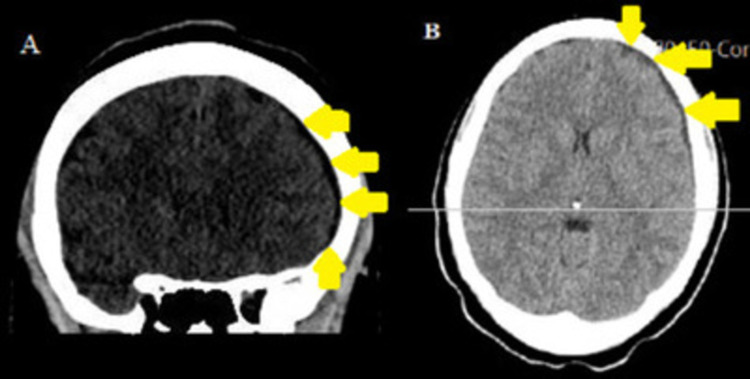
Left subdural hematoma Noncontrast coronal (A) and axial (B) CT scan of the brain demonstrating 4 mm thick subdural hematoma over the left frontal convexity (yellow arrows). This hematoma appears isodense to hypodense which is a characteristic of subacute subdural hematoma.
